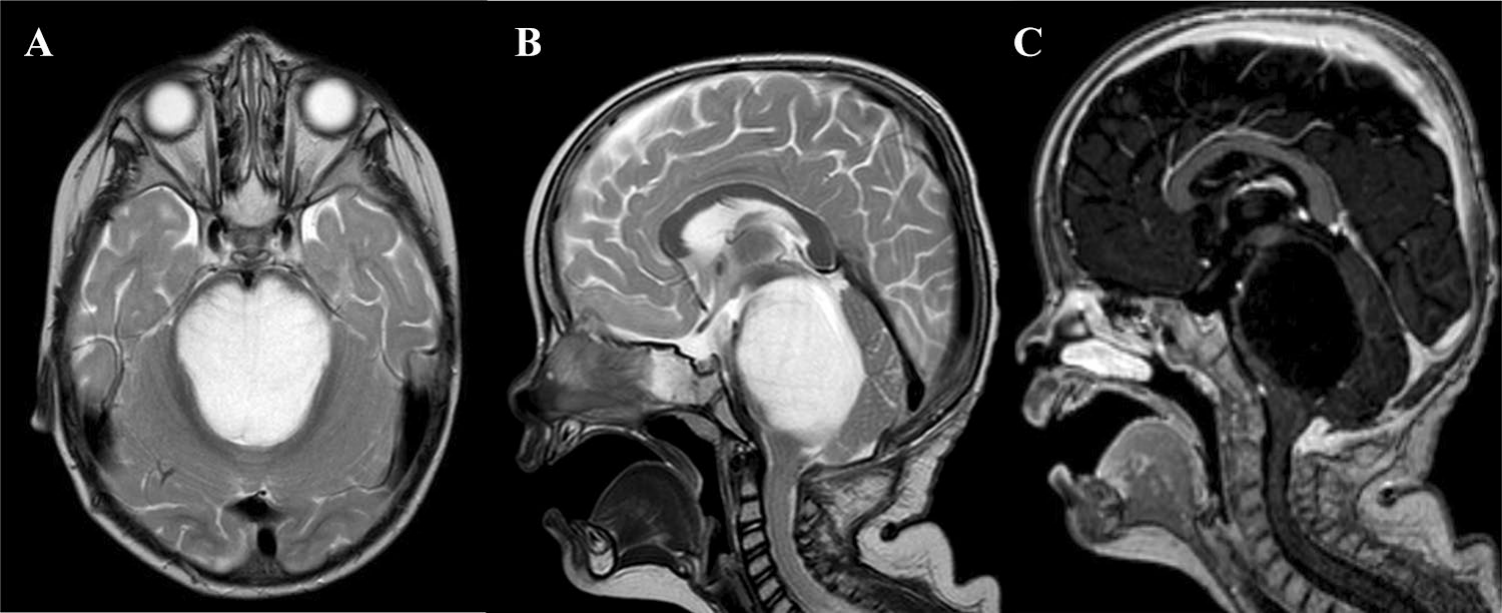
Diagnostic MRI of large pontine tumor. A T2-weighted axial images demonstrating pons involvement (over 66% of the diameter). B T2 sagittal sequence. C T1 sagittal after gadolinium administration displaying hypointense contrast non-enhancing tumor
